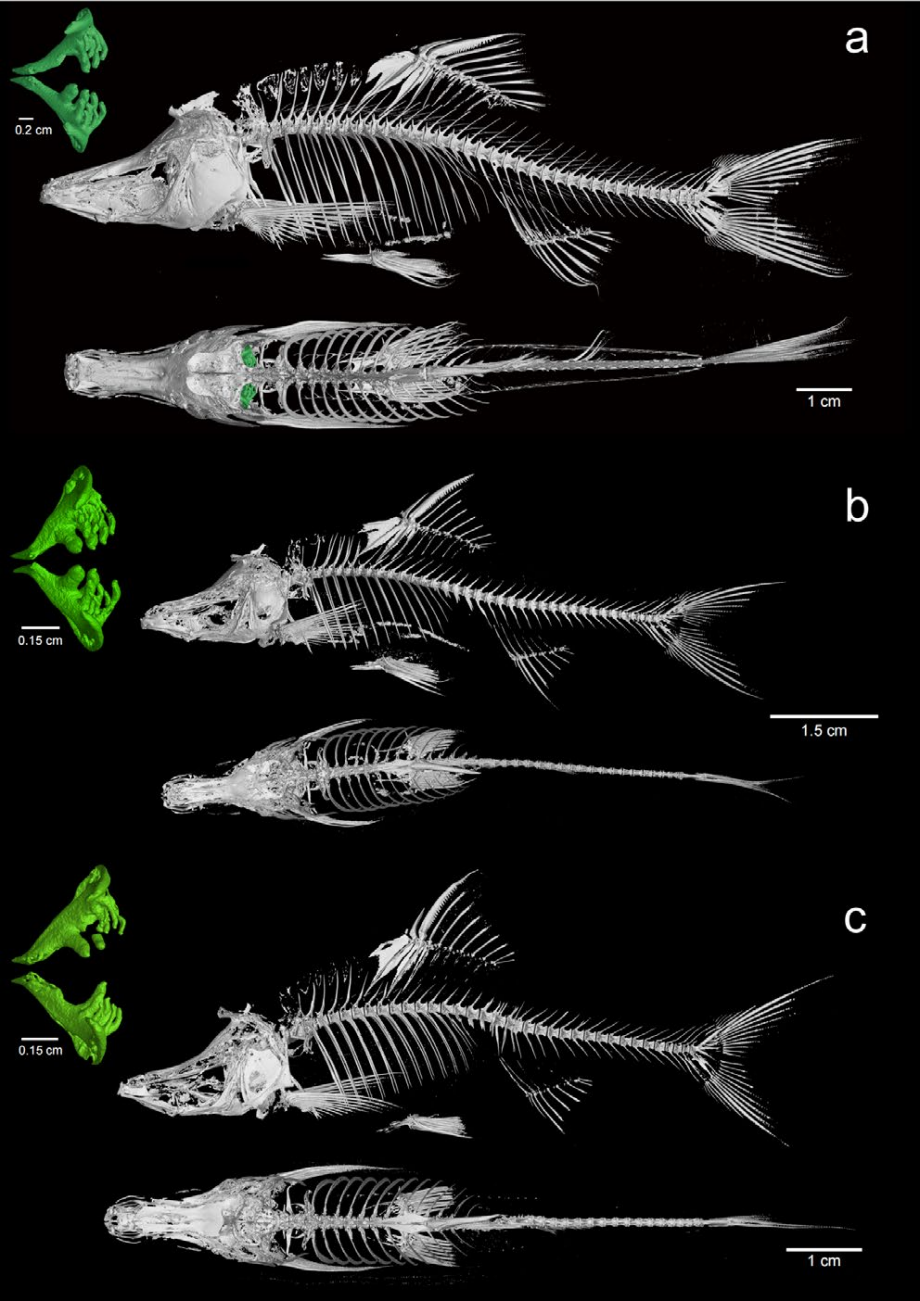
Micro‐CT graph and reconstructed pharyngeal dentition. (a) *Sinocyclocheilus changlensis*, new species; (b)
